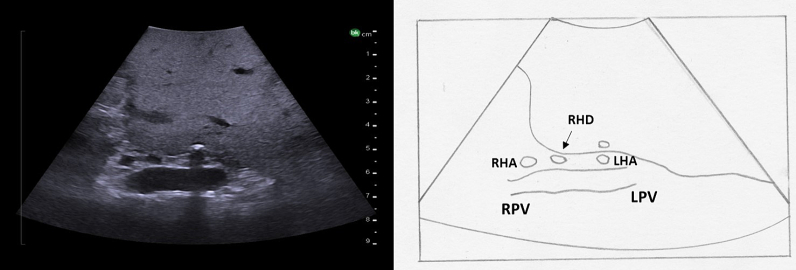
Ultrasound image demonstrating the bifurcation of the proper hepatic artery into the left and right hepatic arteries. RPV right portal vein, LPV left portal vein, RHA right hepatic artery, RHD right hepatic duct, LHA left hepatic artery.
